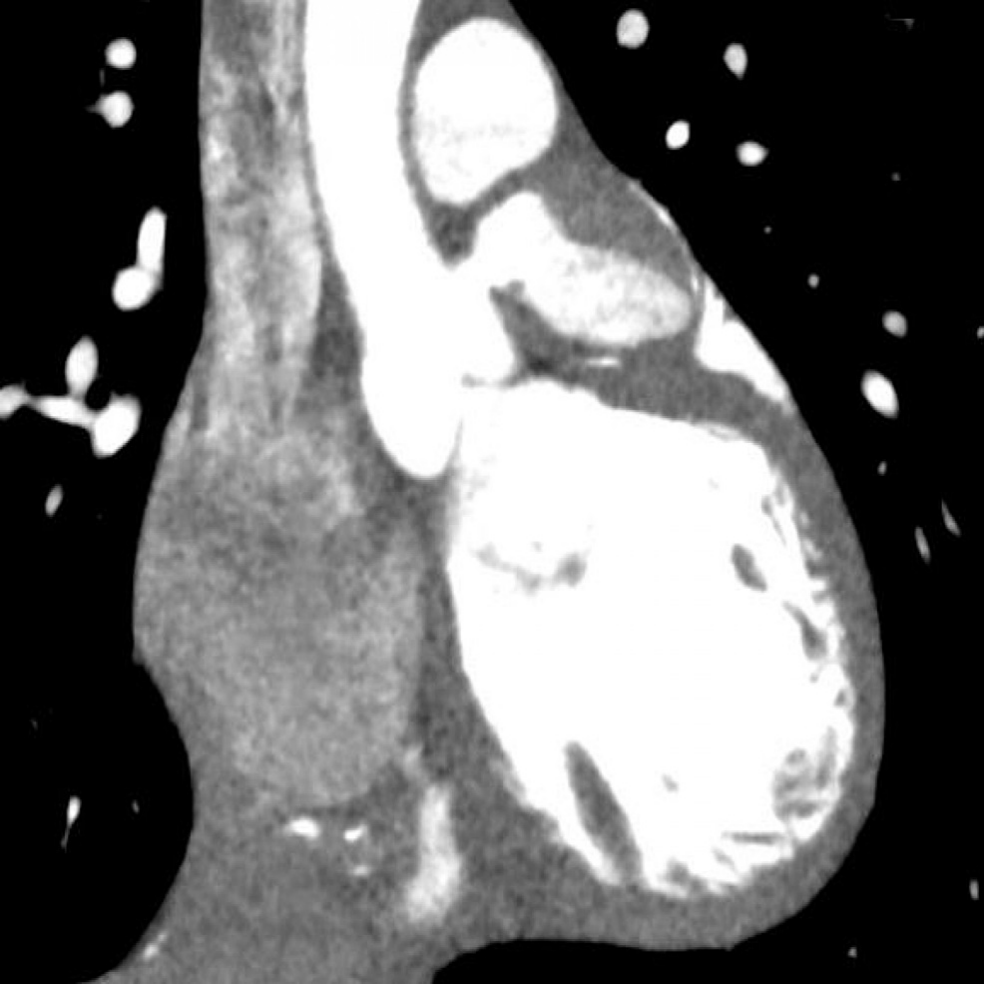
CTA demonstrating a giant saccular aneurysm of the left main coronary artery CTA, Computed tomography angiography.
